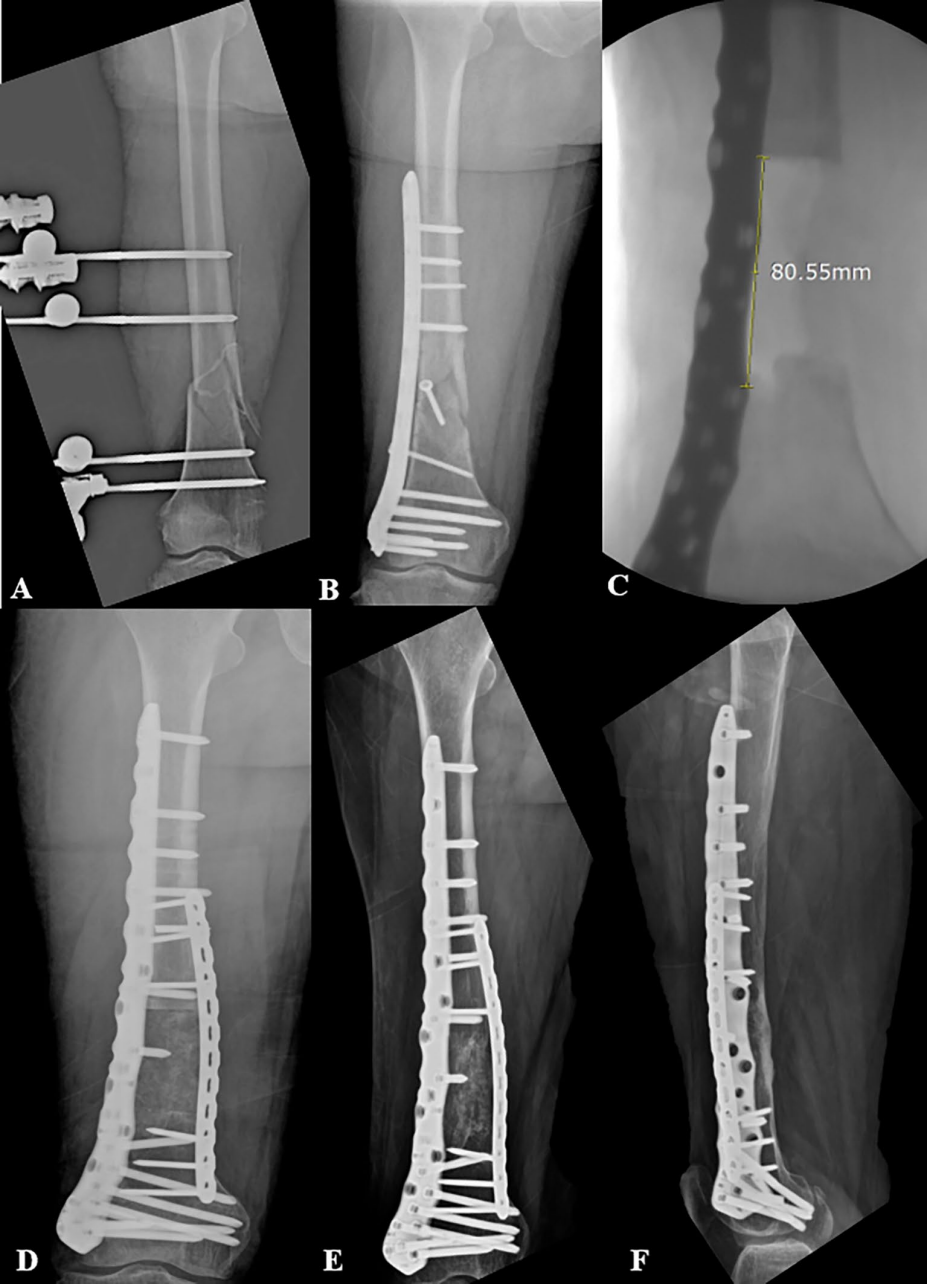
(A), (B) A 64-year-old female patient had a Gustilo-Anderson type III B open fracture of the distal 1/3 femur due to a pedestrian traffic accident. Initial management with temporary external fixation, followed by open reduction and internal fixation was performed at a local clinic. Six months after the surgery, no callus formation was observed. After examination, osteomyelitis was diagnosed, and the patient was referred to our hospital for further management. (C), (D) Serial debridement was performed until the infection was controlled. After infection control, the iliac strut bone was grafted onto the defect area with additional screw and plate fixation. The bone defect was measured to be 8.0 cm. (E), (F) Anteroposterior and lateral plain radiographs taken 24 months postoperatively. The patient achieved an uneventful complete bone union.
